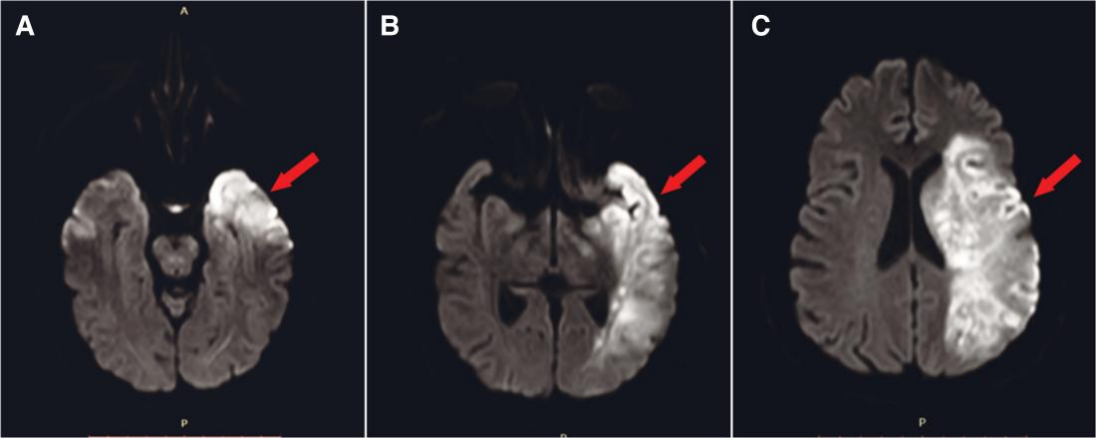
Brain MRI findings. Diffusion-weighted magnetic resonance imaging of the brain revealed increase in signal intensity in the left temporal (*A*, *B*), and parietal lobes (*C*), consistent with an acute infarct in the territory of the left middle cerebral artery. Arrows indicate the affected regions.
